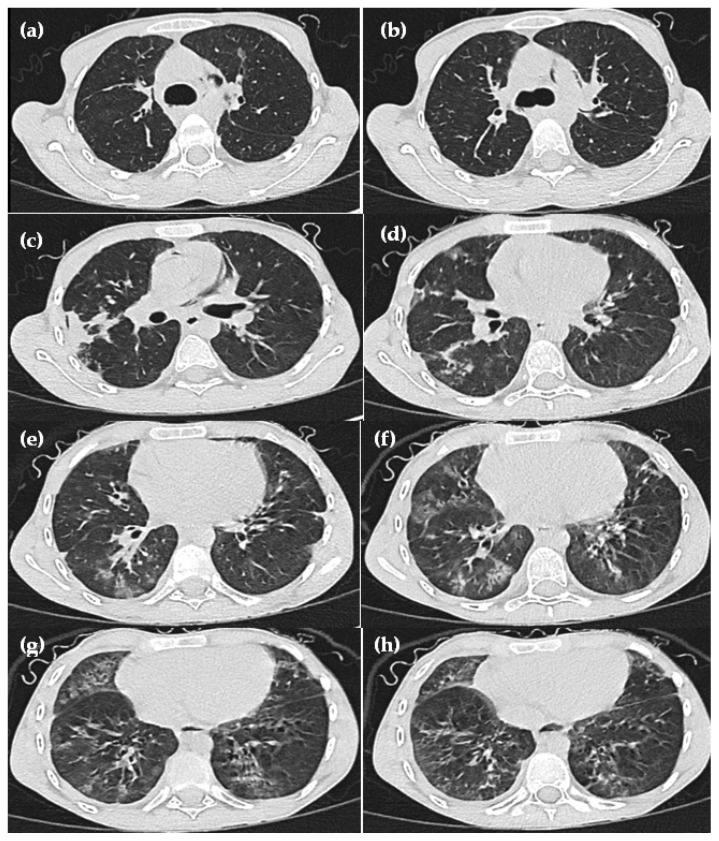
Chest computed tomography (CT) in an adolescent with ataxia–telangiectasia demonstrating multiple cylindrical bronchiectases in both lung fields (a–h), small diffuse ground-glass opacities and pseudonodular alveolar consolidations, predominantly peripheral and basal, mainly in the right lung (c,d). A “tree-in-bud” pattern is seen in the right lower lobe (f–h).
